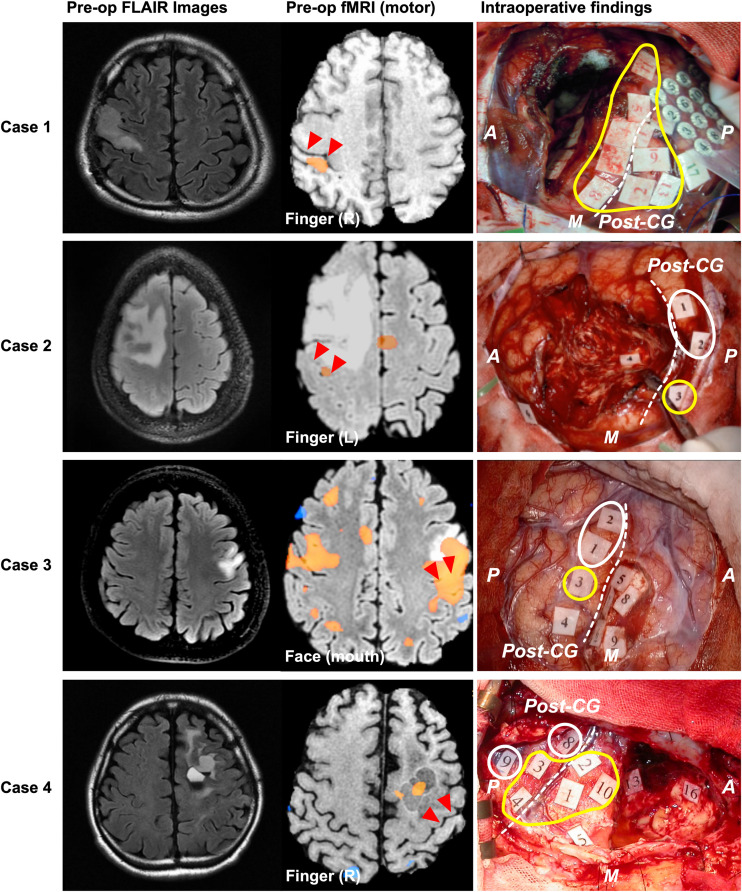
Patients whose motor functions shifted in Type 1. The left, middle, and right columns show the preoperative FLAIR images, preoperative functional MRI for movement, and intraoperative findings, respectively. In Cases 1 and 2, blood oxygenation level dependency (BOLD) was observed in the postcentral gyrus during the movement task. However, in Case 4, BOLD dependency was observed within the resection cavity of previous surgery, but not in the precentral or postcentral gyrus. Positive mapping sites for movement were found in the postcentral gyrus of four patients during awake surgery. In Cases 1 and 4, positive motor responses were elicited in both the precentral and the postcentral gyri. However, in Cases 2 and 3, positive motor responses were only found in the postcentral gyrus. The red triangles in the middle column and white broken line in the right column indicate the central sulcus; the yellow circles indicate positive mappings for motor function sites; the white circles indicate positive mappings for sensory sites. A: anterior; P: posterior; M: medial side; CG: central gyrus. Here, we show Tags and symptoms elicited by electrical stimulation except for sensory and motor. Case 1: Tag 17, sensory; Case 2: Tag 4, negative motor response (foot); Tag 6, emotion recognition; Case 3: Tags 4, 6, and 7, dysarthria; Tags 5 and 9, anarthria; Tag 8, negative motor response; Case 4: Tags 5, 13, and 16, paraphasia.
